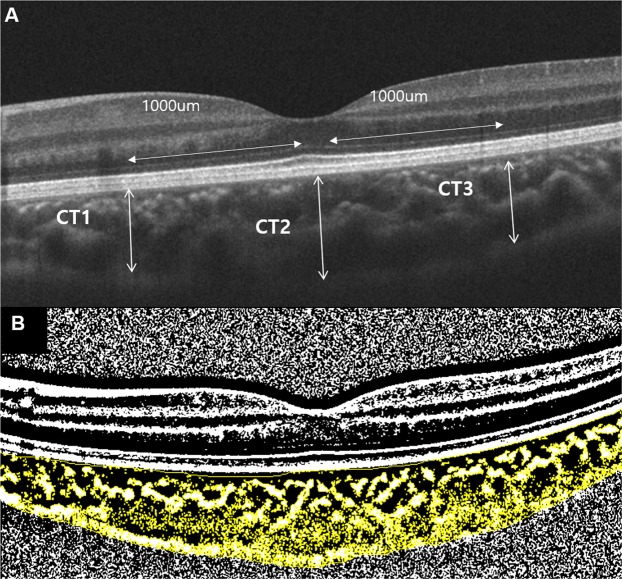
Measurements of choroidal thickness and vascularity. (A) Nasal parafoveal choroidal thickness (CT1), subfoveal choroidal thickness (CT2), and temporal parafoveal choroidal thickness (CT3) were obtained using enhanced depth image optical coherence tomography. CT was measured as the distance between Bruch’s membrane and the choroid-scleral interface. (B) The subfoveal choroidal area with a width of 3 mm, centered at the fovea, was selected. Using ImageJ software, the image was binarized with Niblack’s method, and the ratio of vascular area (black pixels) to stromal area (white pixels) was quantified.
